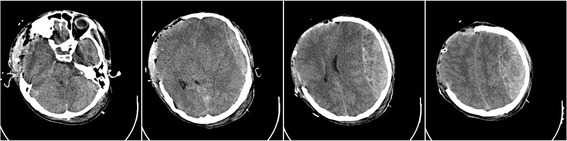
Computed tomography following the first decompressive surgery showing contralateral delayed epidural hematoma
